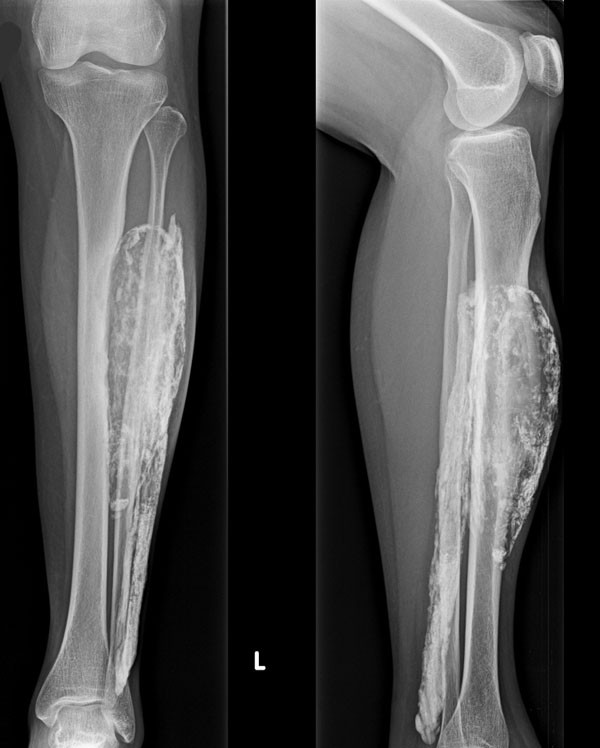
Initial anterior-posterior and lateral plain radiographs of left leg.
